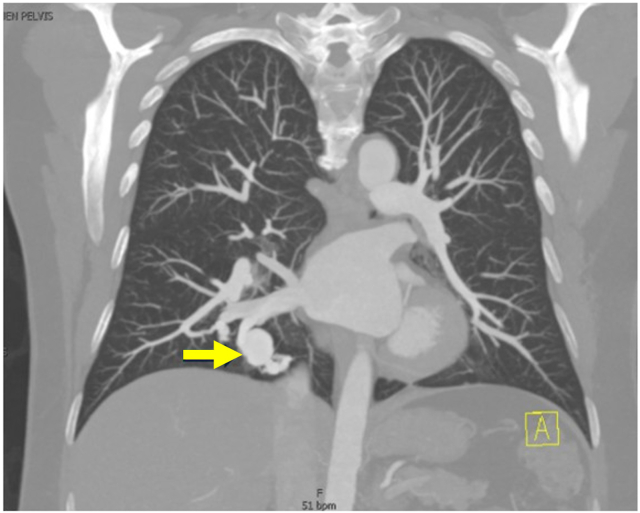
Chest CT angiography, maximal intensity projection, coronal display, demonstrates dilated peripheral pulmonary blood vessels in the right lower lobe *(arrow)* suggestive of a PAVM.
